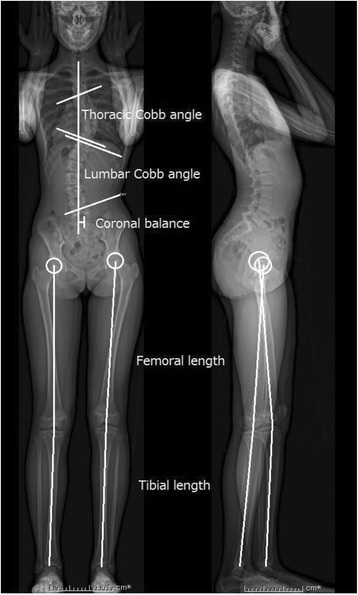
Measurements obtained using whole-body EOS radiography in the upright position. Thoracic Cobb angle, lumbar Cobb angle, and coronal balance were assessed in the coronal plane obtained from whole-body EOS radiography. Structural leg length discrepancy was defined as the difference between the sums of the bilateral femoral and tibial lengths obtained from three-dimensional distance using the EOS system
